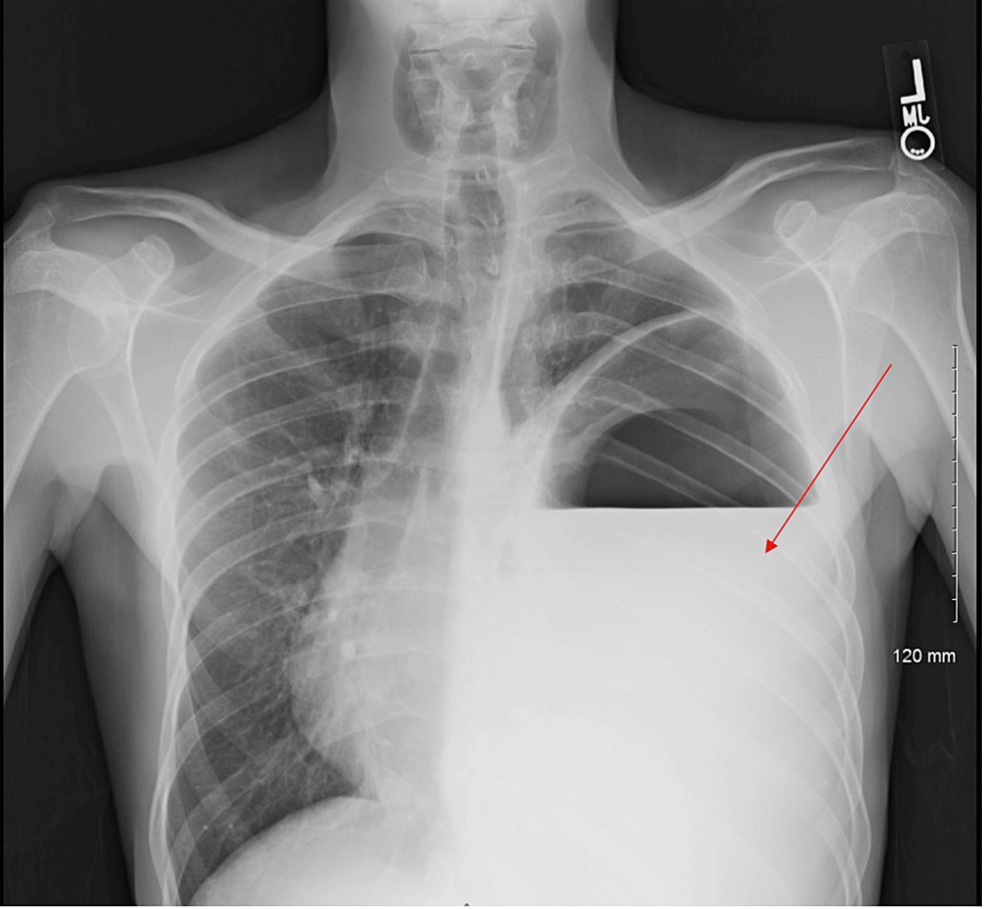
Chest X-ray image A significant air-fluid level (red arrow) is seen in the left hemithorax representing a large loculated pleural effusion/Hydro pneumothorax.
